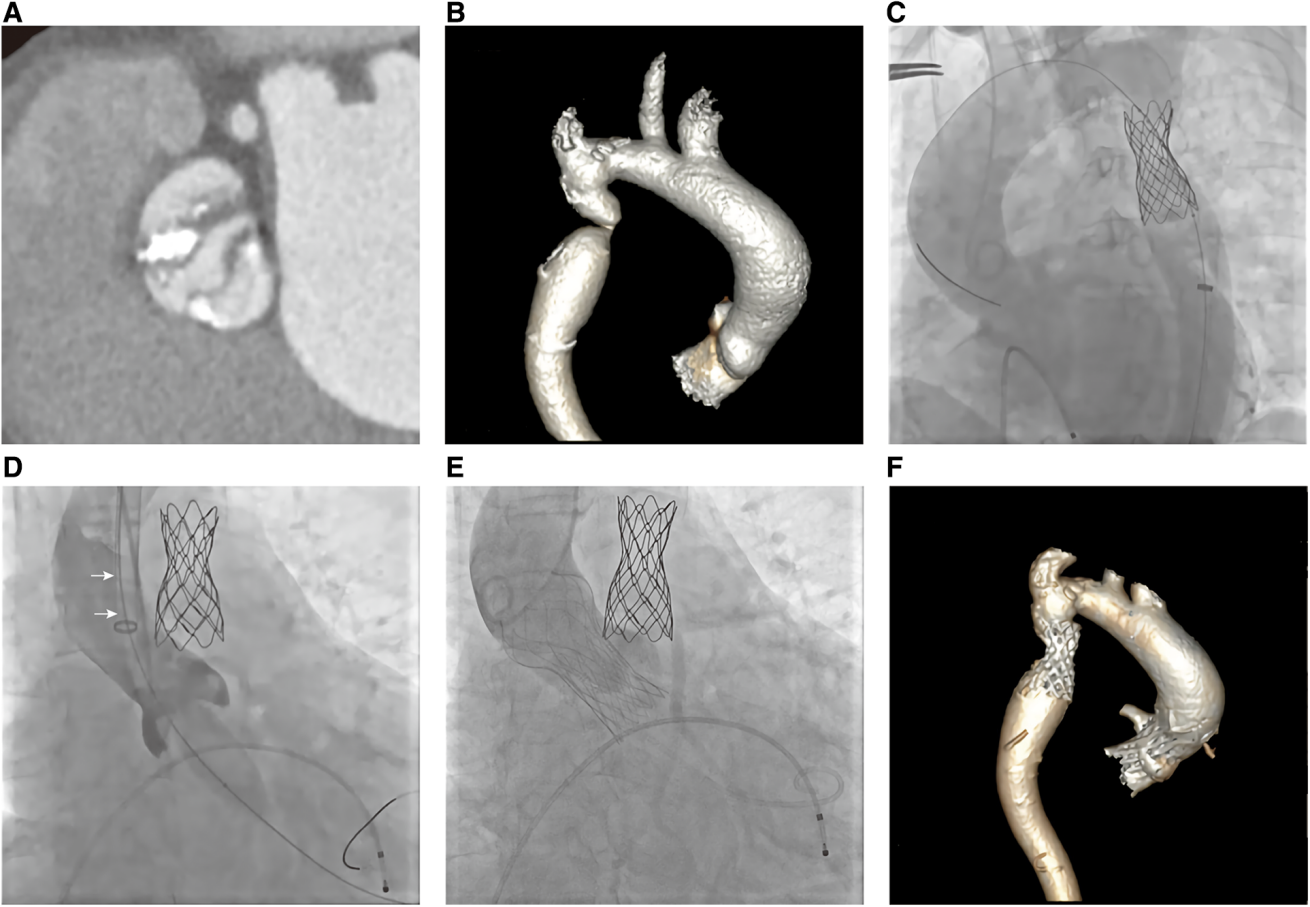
Ct aortogram and invasive aortic angiography. (A, B) CTA showed severe calcified bicuspid aortic valve stenosis (type 0) and stenotic calcified eccentric aortic coarctation 20 mm distal to the left subclavian artery; (C)A CP stent was implanted *via* the right femoral artery; (D) Aortic valve balloon dilatation *via* the left carotid artery (arrow: 20F sheath); (E) Prosthetic valve was implanted; (F) CT follow-up revealed both devices were properly positioned.
